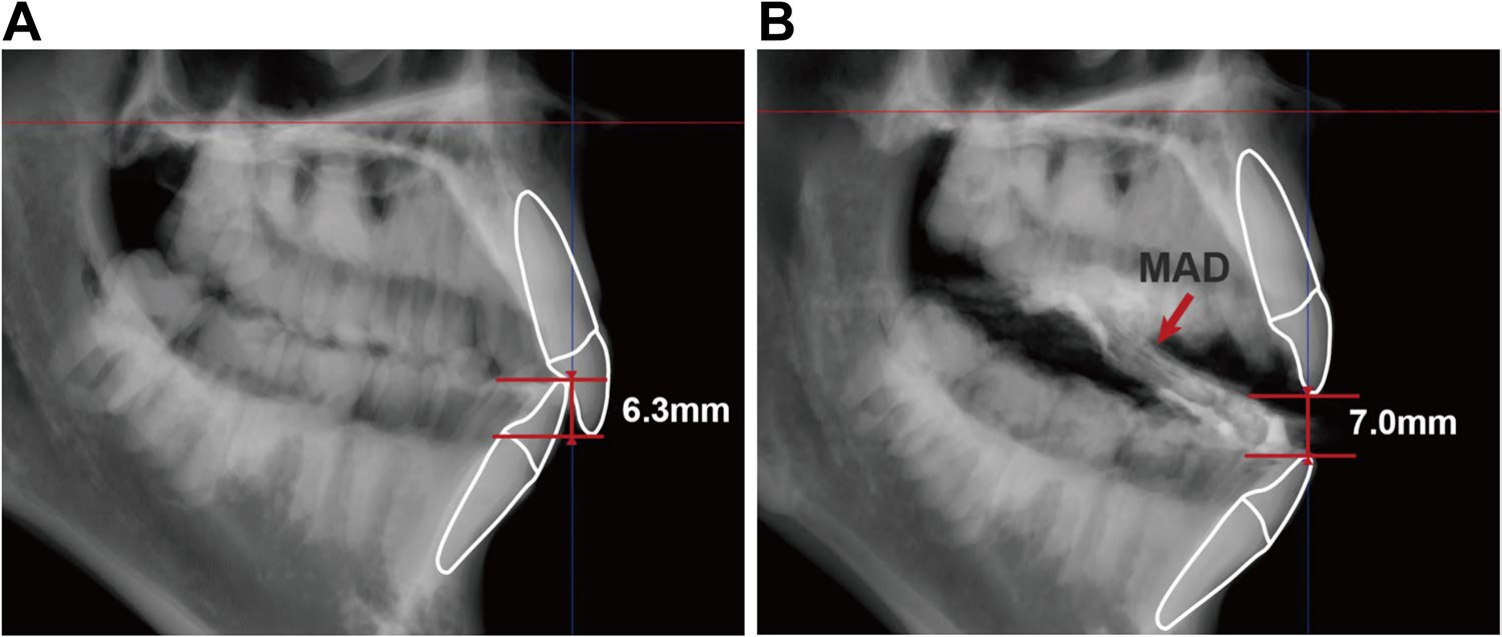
Measurements of mandibular vertical opening using cone beam computed tomography (CBCT) imaging. (A) Measurement of overbite (6.3 mm in this example). (B) Measurement of the vertical distance between the tip of upper and lower incisors with MAD in situ (7.0 mm in this example). In this case, the vertical opening with MAD in situ is 13.3 mm (i.e., sum of the 6.3 mm and 7.0 mm)
